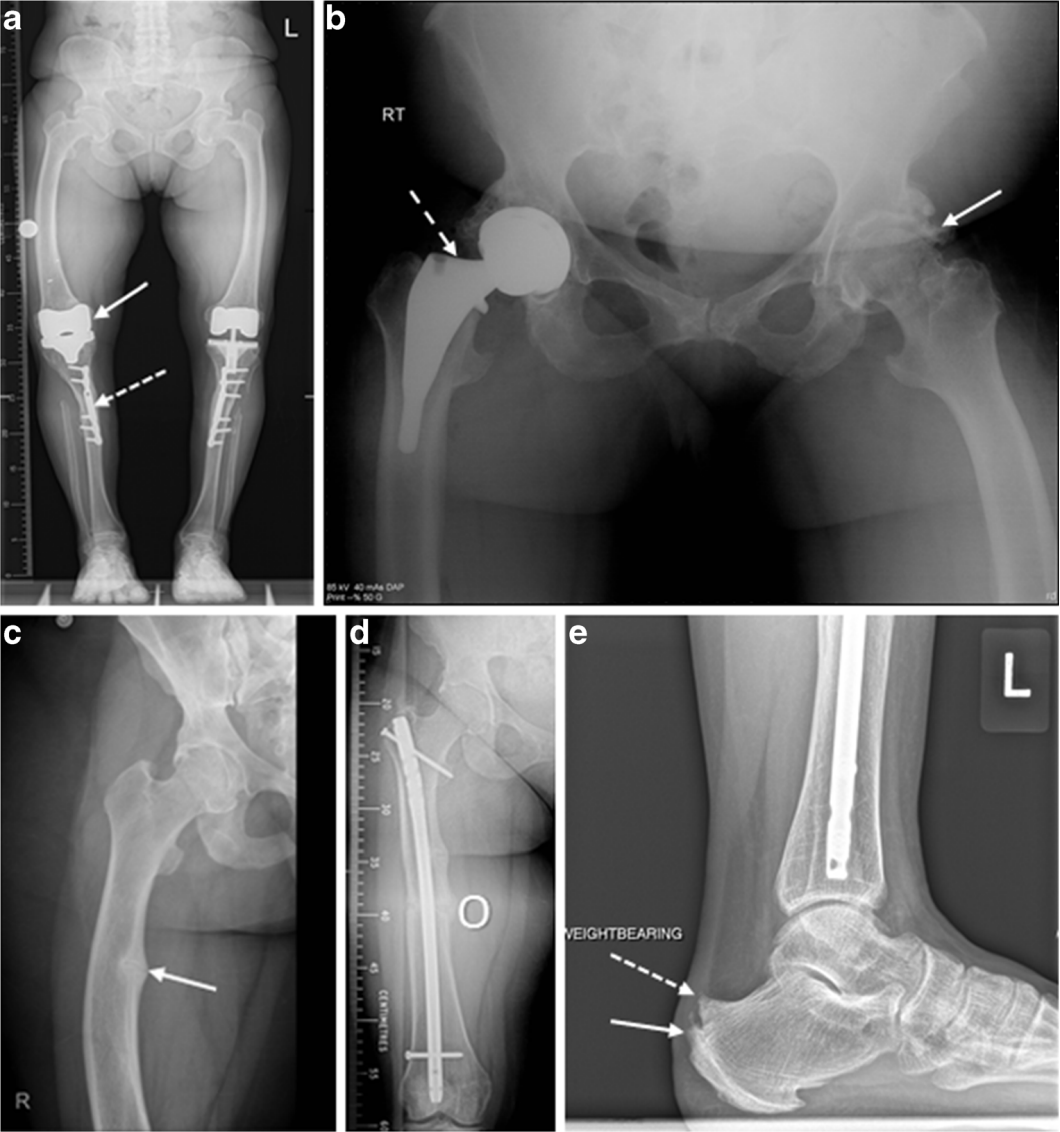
Orthopaedic complications of XLH. a Standing antero-posterior limb alignment radiograph of a 75 years old female demonstrating bilateral bowed coxa vara deformity of the proximal femur with hip osteoarthritis, femoral shaft bowing, bilateral total knee replacements (solid arrow), tibial shaft plates from osteotomies in adolescence (dashed arrow) and ankle arthritis with loss of joint space. b Antero-posterior pelvic radiograph of a 60 years old female demonstrating left hip osteoarthritis showing loss of joint space and osteophytes (solid arrow) and a right short stem un-cemented total hip replacement (dashed arrow). c-d Antero-posterior radiograph of the right femur of a 33 years old female with a Looser’s zone of the medial cortex of the femoral shaft (solid arrow) treated with a femoral shaft osteotomy (O) and intra-medullary rod fixation to realign the bone shape and aid fracture healing. e Lateral standing ankle radiograph of a 32 years old female showing a prominent posterior/superior corner Haglund’s deformity of the os calcis (dashed arrow) and insertional Achilles’ tendon bony spur enthesophytes (solid arrow)
